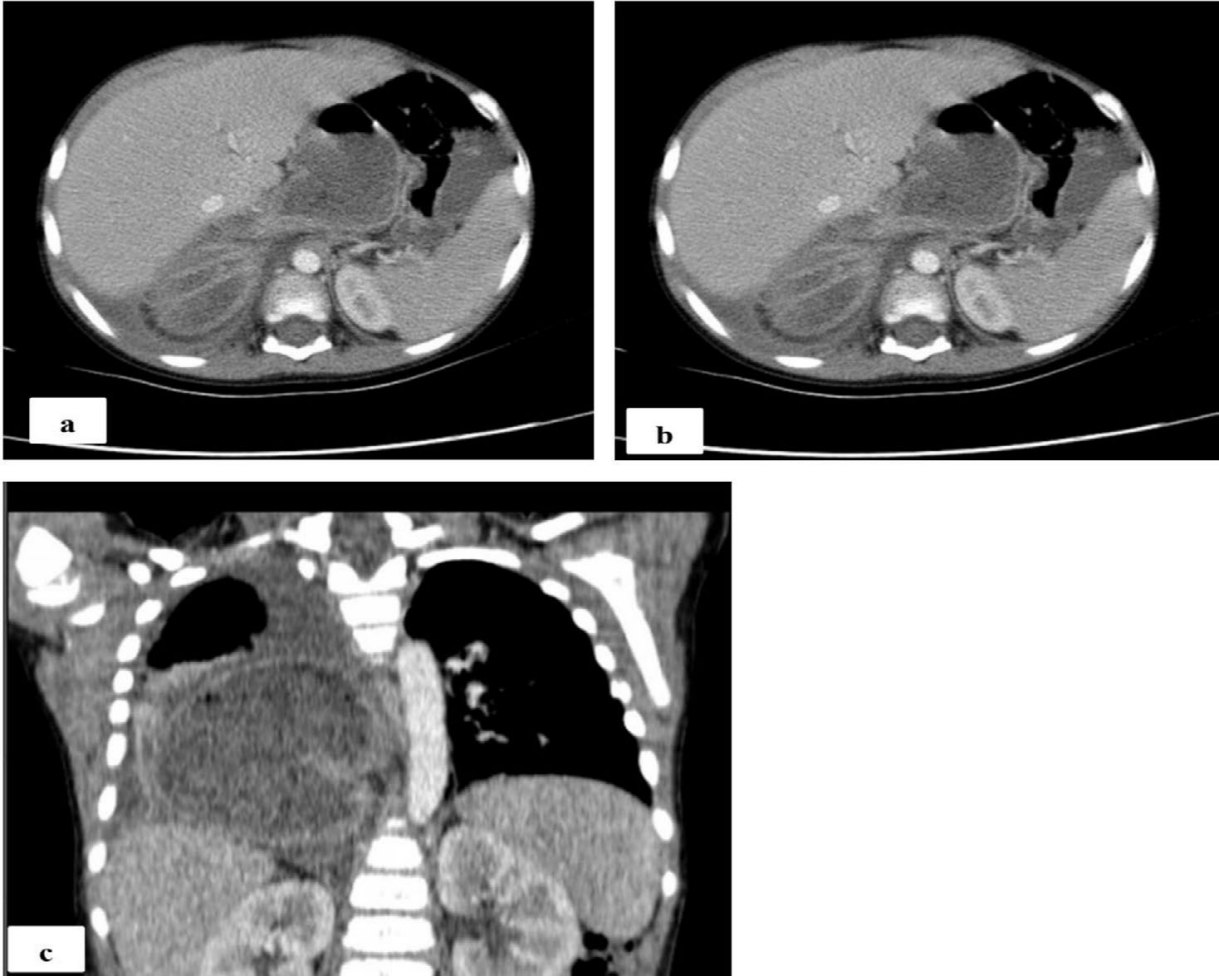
CT scan findings. (a) Contrast enhanced axial chest CT showing large trans‐hiatial herniation of the proximal stomach to the level of the gastric mid body, herniated stomach occupying the lower posterior mediastinum and the right lower thoracic cavity. (b) Contrast enhanced chest CT showing large trans‐hiatial herniation of the proximal stomach to the level of the gastric mid body with incomplete gastric volvulus. (c) Coronal reconstruction of contrast enhanced chest CT showing the herniated stomach occupying the lower posterior mediastinum and the right lower thoracic cavity.
